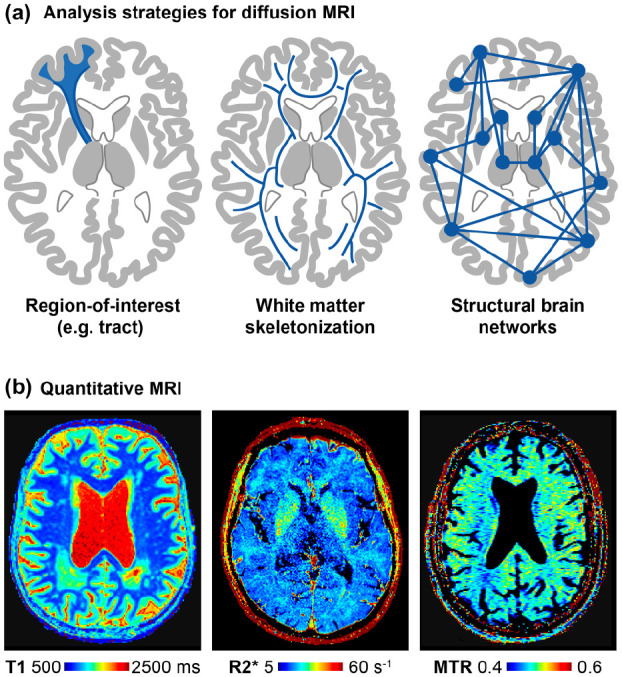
Quantitative MRI: (a) Analysis strategies for diffusion MRI can vary
